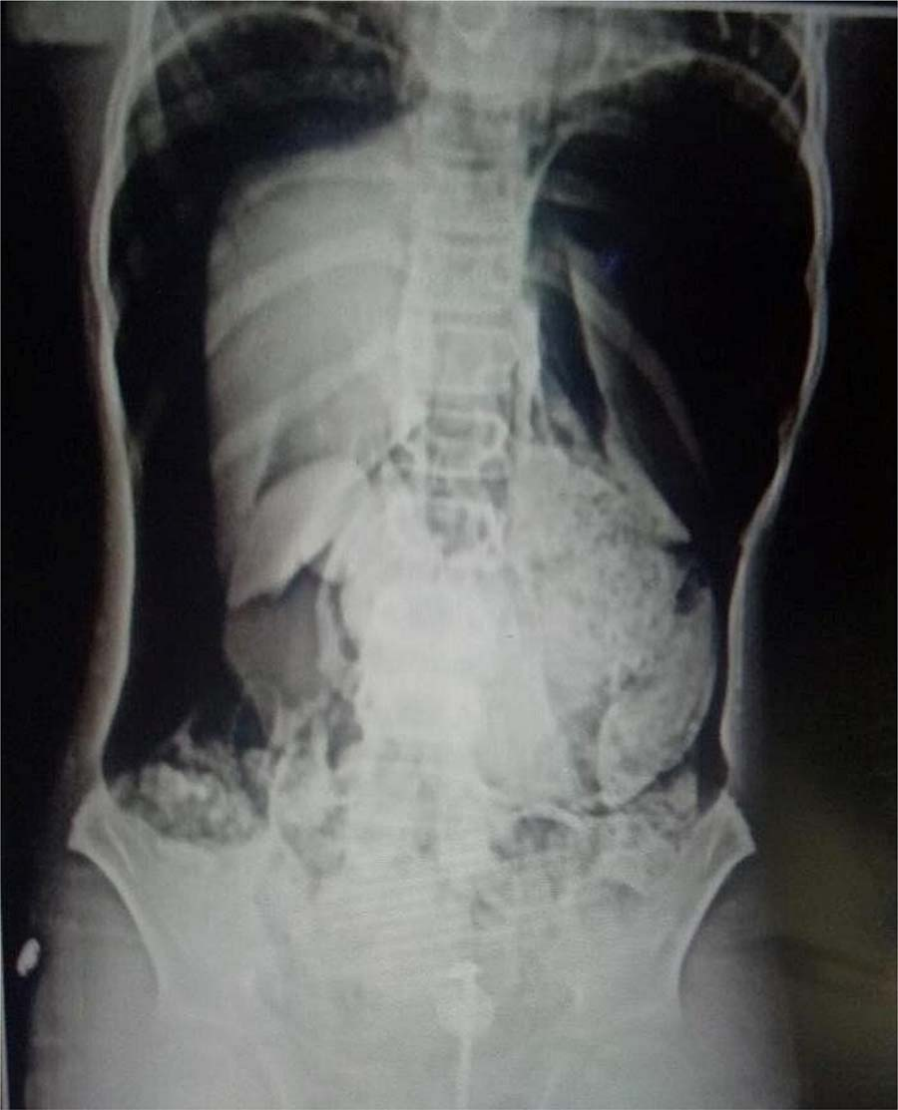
Abdominal X-rays with massive pneumoperitoneum.
